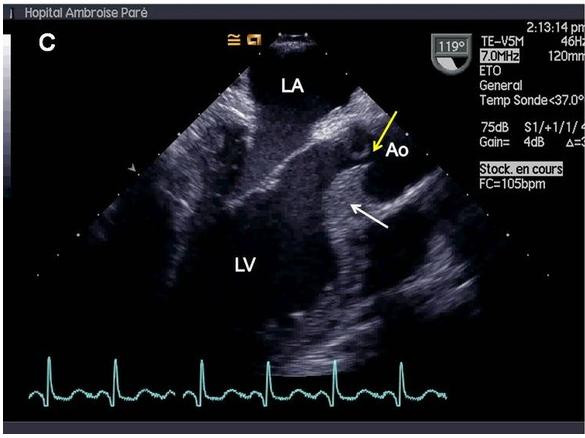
Transesophageal echocardiography showing septal hypertrophy (white arrows) and lack of central coaptation of aortic valve (yellow arrows), resulting in massive aortic regurgitation. Ao = aorta. LA = left atrium. LV = left ventricle.
